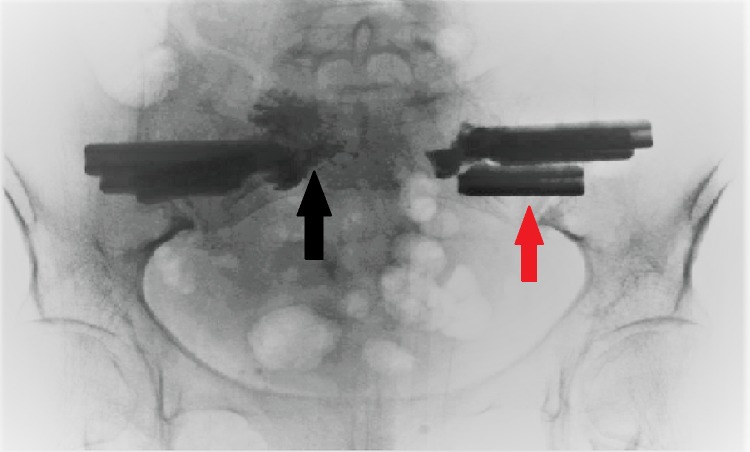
Intraoperative C-arm fluoroscopic radiograph of the pelvis and sacrum The figure shows three triangular implants (red arrow) placed bilaterally through the ilium and sacral ala across the SI joint. Also seen is the polymethylmethacrylate (PMMA) cement (black arrow) inserted into the SI joint bilaterally.
